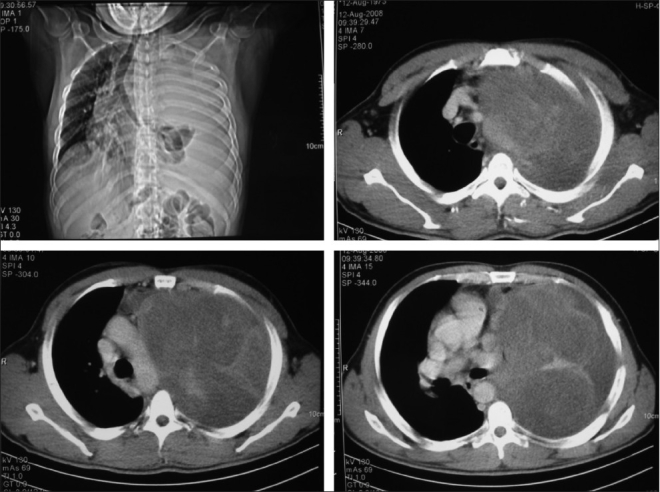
CT scan of the chest — Huge left intrathoracic mass
